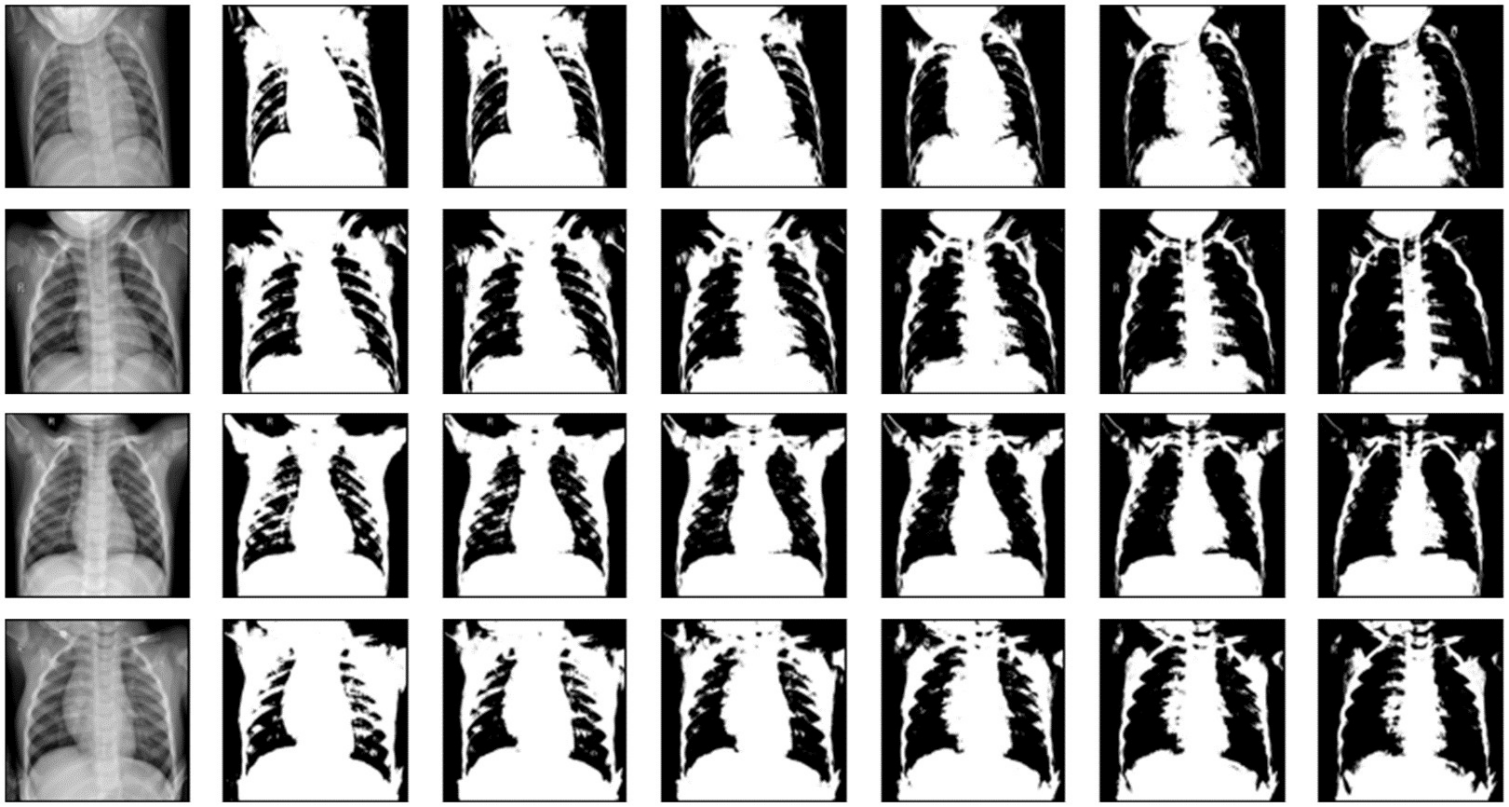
Four normal X-ray images and their corresponding binary images. The original gray-level images are leftmost. Each binary image, from left to right, is generated with threshold values 100, 110, 120, 130, 140, and 150, respectively.
